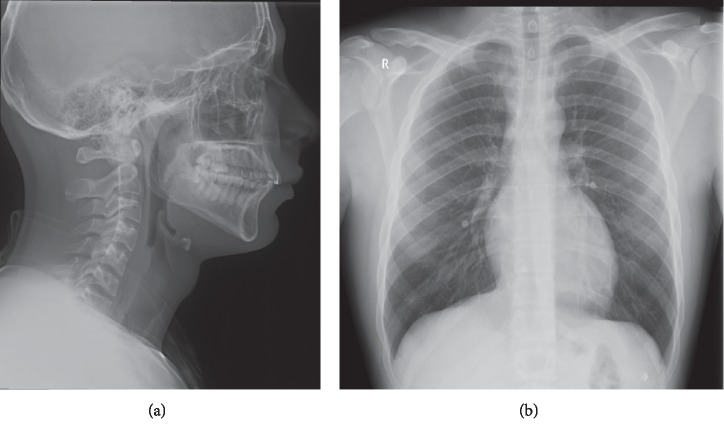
Lateral neck (a) and posteroanterior chest (b) X-rays after 2 weeks showing resolution of previously shown air in the neck and anterior mediastinum.
